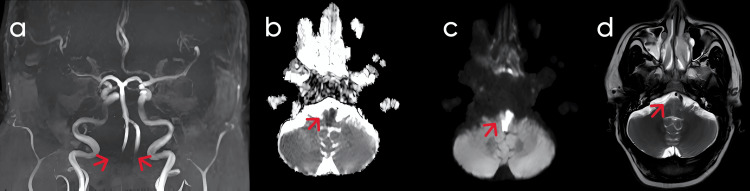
MRI Sequences of Case 2 (a) MR (magnetic resonance) angiography showed flow restriction in both vertebral arteries. (b) ADC (apparent diffusion coefficient) mapping confirmed diffusion restriction, consistent with acute infarction. (c) DWI (diffusion-weighted imaging) revealed areas of restricted diffusion in the affected regions. (d) FLAIR (fluid-attenuated inversion recovery) imaging showed hyperintensities in the medial aspect of the bilateral medulla oblongata.
